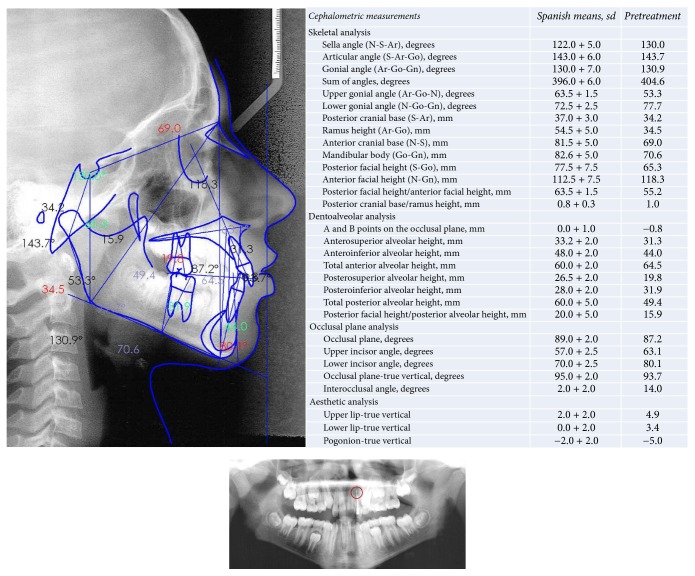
Panoramic radiograph, lateral radiographic records, and cephalometric measurements.
